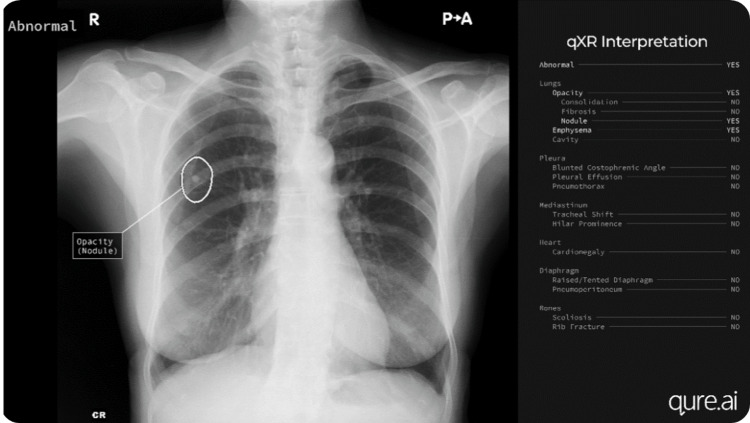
X-ray images with the use of Qure.AI software in lung nodules Image credits: Permission was obtained from the original publisher to adapt this image [29].
